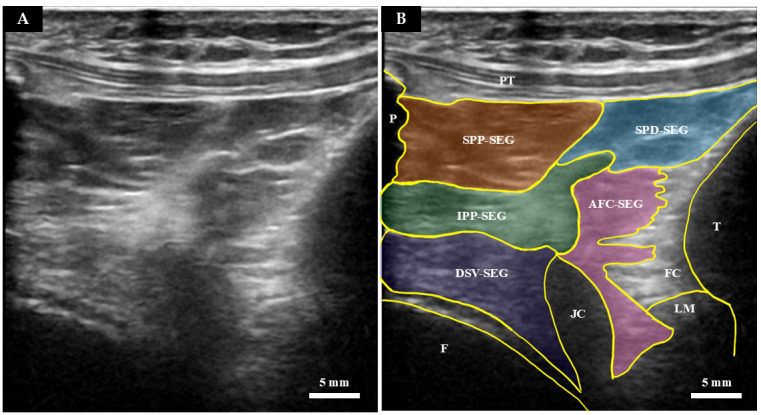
Long-axis ultrasound image of a Thiel-embalmed IPFP. (A) Original ultrasound image. (B) Color-coded representation of the segmental divisions. P: patella; PT: patellar tendon; T: tibia; F: femur; JC: joint cavity; LM: lateral meniscus; FC: fibro-capsular complex.
